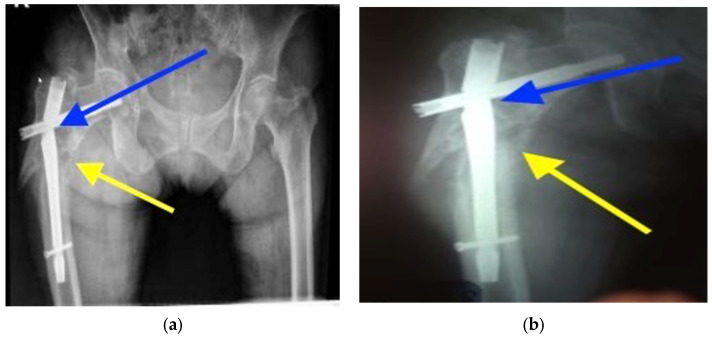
(a) Radiographic aspect of the damaged implant (blue arrow) and of the nonunion (yellow arrow). (b) Radiographic aspect of the damaged implant (blue arrow) and of the nonunion (yellow arrow).
